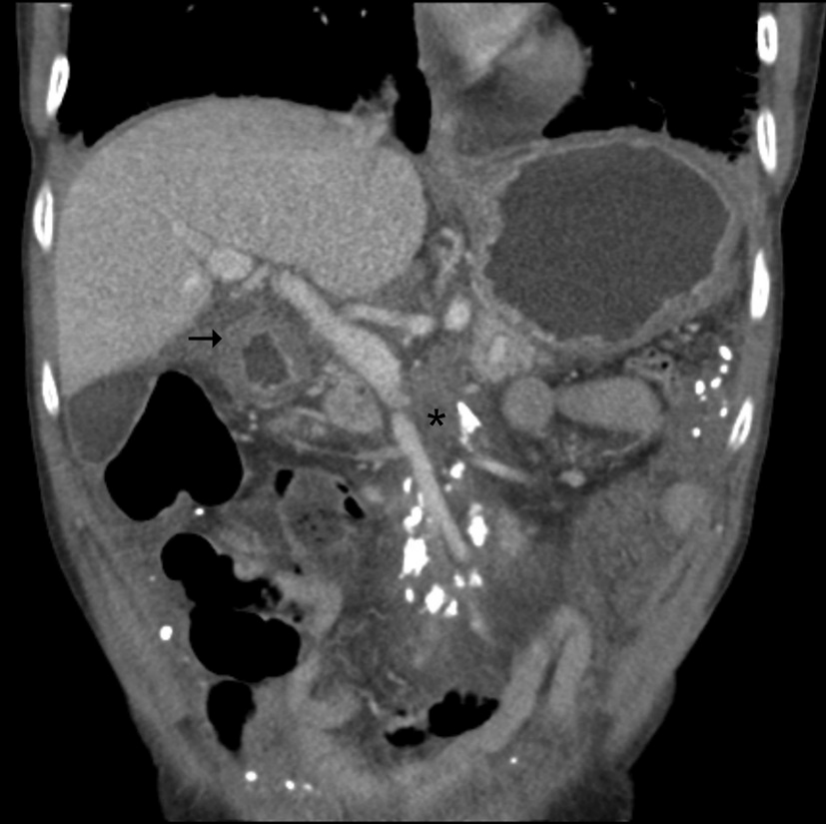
Coronal reconstruction of CT scan of the abdomen. Abnormal soft tissue with coarse calcified deposits centred by the mesenteric root (star) and a thick-walled first part of the duodenum (arrow).
